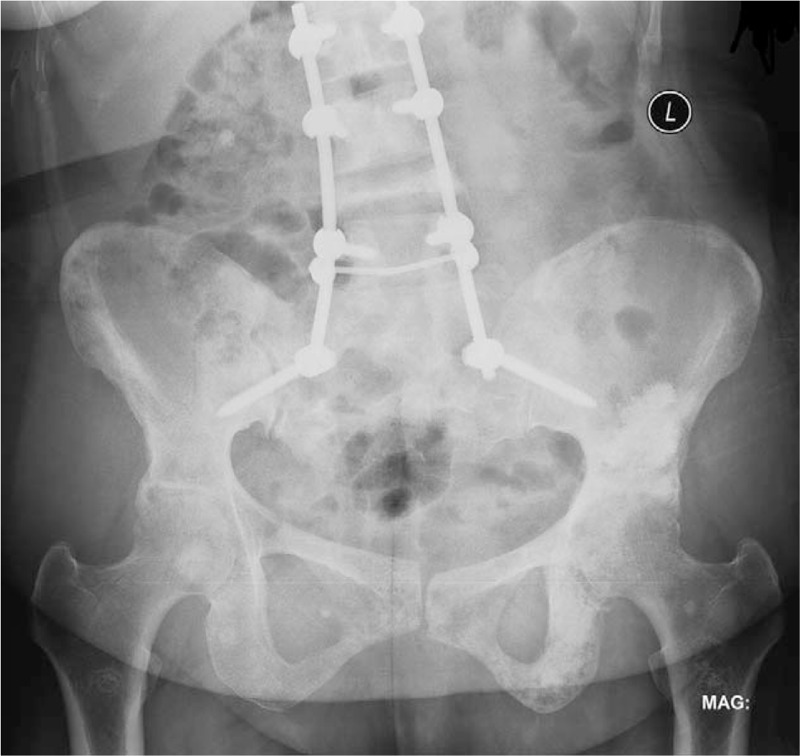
The patient underwent further reconstructive operation on lumbar metastatic lesion 12 months after RFA-PA procedure in her left acetabulum, however, antero-posterior pelvic radiographs taken at 36 months followup did not show any change at the area of cement augmentation (patient 25). PA = percutaneous acetabuloplasty, RFA = radiofrequency ablation.
